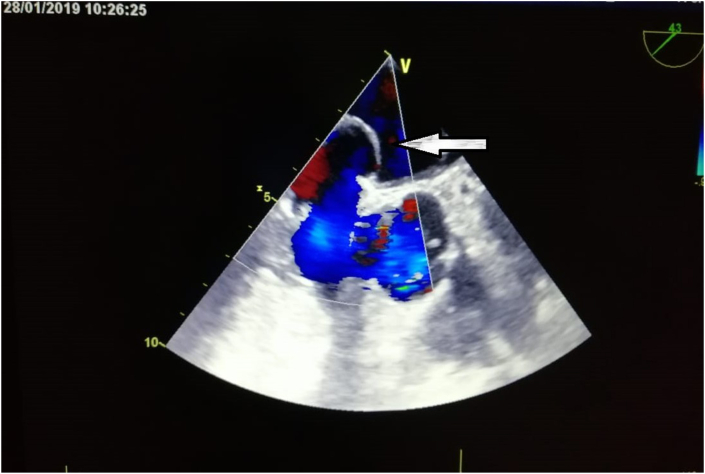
Transoesophageal echocardiography demonstrated an ASIA.
